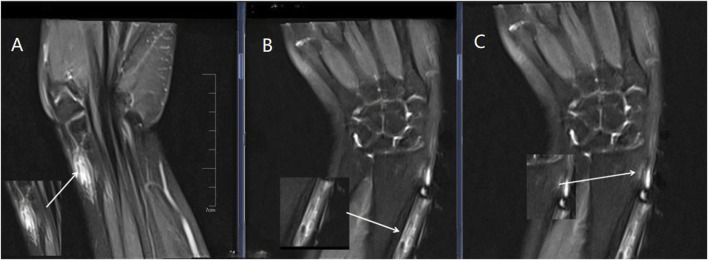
Wrist MRI findings. (A) Soft tissue edema on the radial side of the left wrist. (B) Soft tissue edema on the radial side of the right wrist. (C) Mild bone marrow edema in the distal right radius.
